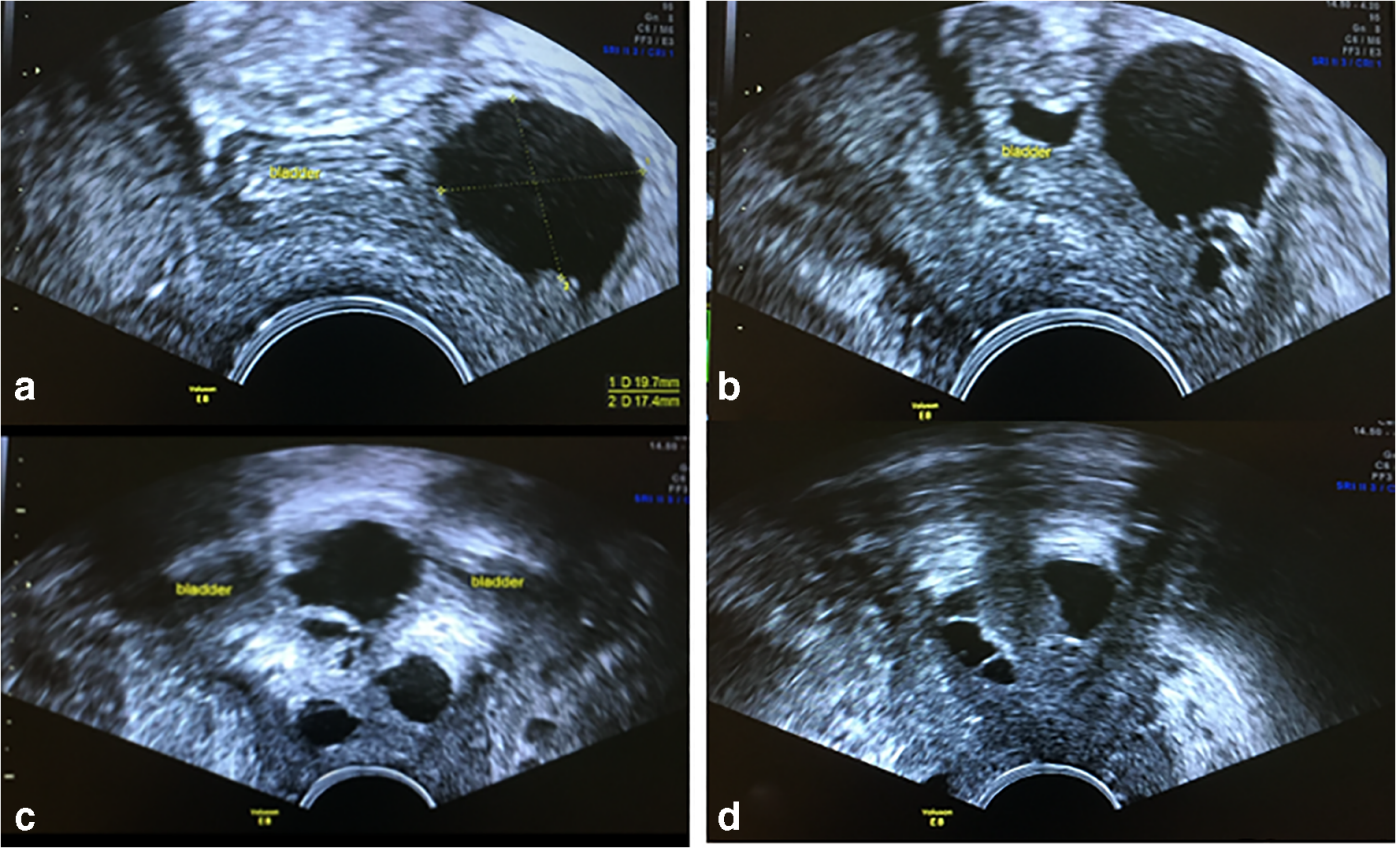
Transvaginal ultrasound showing cystic lesions (diverticulum) in the bladder wall and around the urethra. a Mid-sagittal image shows a cystic portion of the diverticulum in the bladder wall over the trigone. b Smaller cystic lesions inferior to the larger cystic structure are shown at the trigone. c Coronal images show multiple cystic lesions at the base of the bladder and along the proximal urethral length. The bladder is empty. d Coronal section showing cystic structures in the distal urethra
